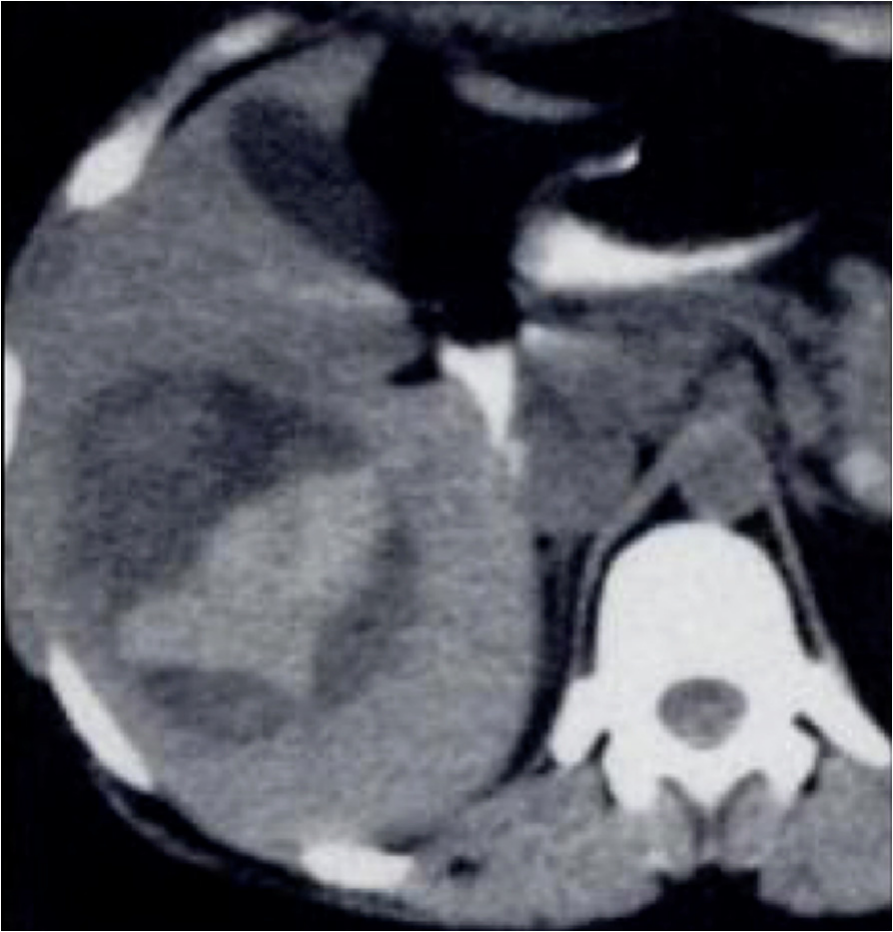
A 48-year-old man with hepatocellular carcinoma. Axial unenhanced CT image of the abdomen showing a hepatic mass on the right lobe with hyperattenuating components suggesting acute bleeding.
